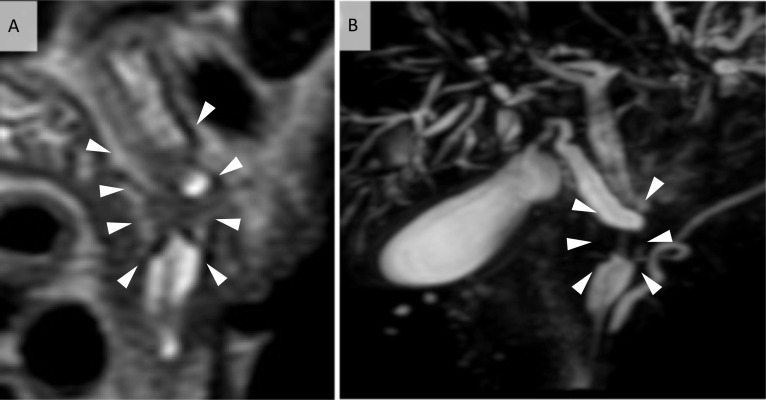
MRI. A Coronal section of T2-weighted imaging showing an irregular signal defect in the middle bile duct (arrowheads). B Magnetic resonance cholangiopancreatography showing mild dilation of proximal bile duct (arrowheads)
